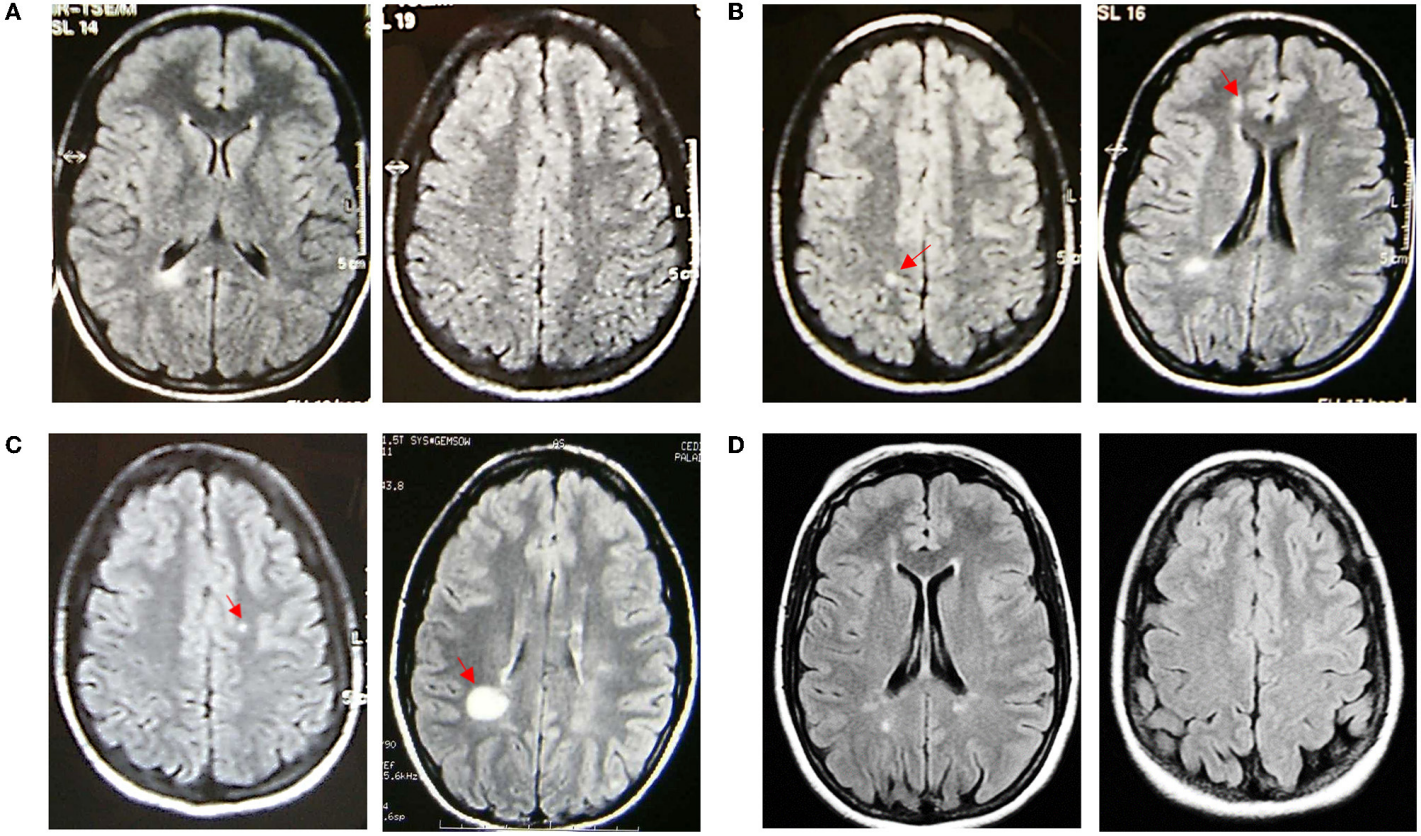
Fluid attenuated inversion recovery axial sequences of MRI scans performed in the patient over time. (A) Baseline MRI on 11/07/2002; (B) MRI on the left (10/01/2003) with a new parietal right lesion (red arrow) (new cortical frontal lesion not shown), MRI on the right (12/09/2003) with a new frontal periventricular right lesion (red arrow) (left periventricular and left temporoparietal junction new lesions not shown); (C) MRI on the left (29/11/2004) showing a new left corona radiata lesion (other three new lesions not shown), MRI on the right (20/05/2005) showing a new supratrigonal right lesion (red arrow); and (D) last MRI on 15/01/2021.
